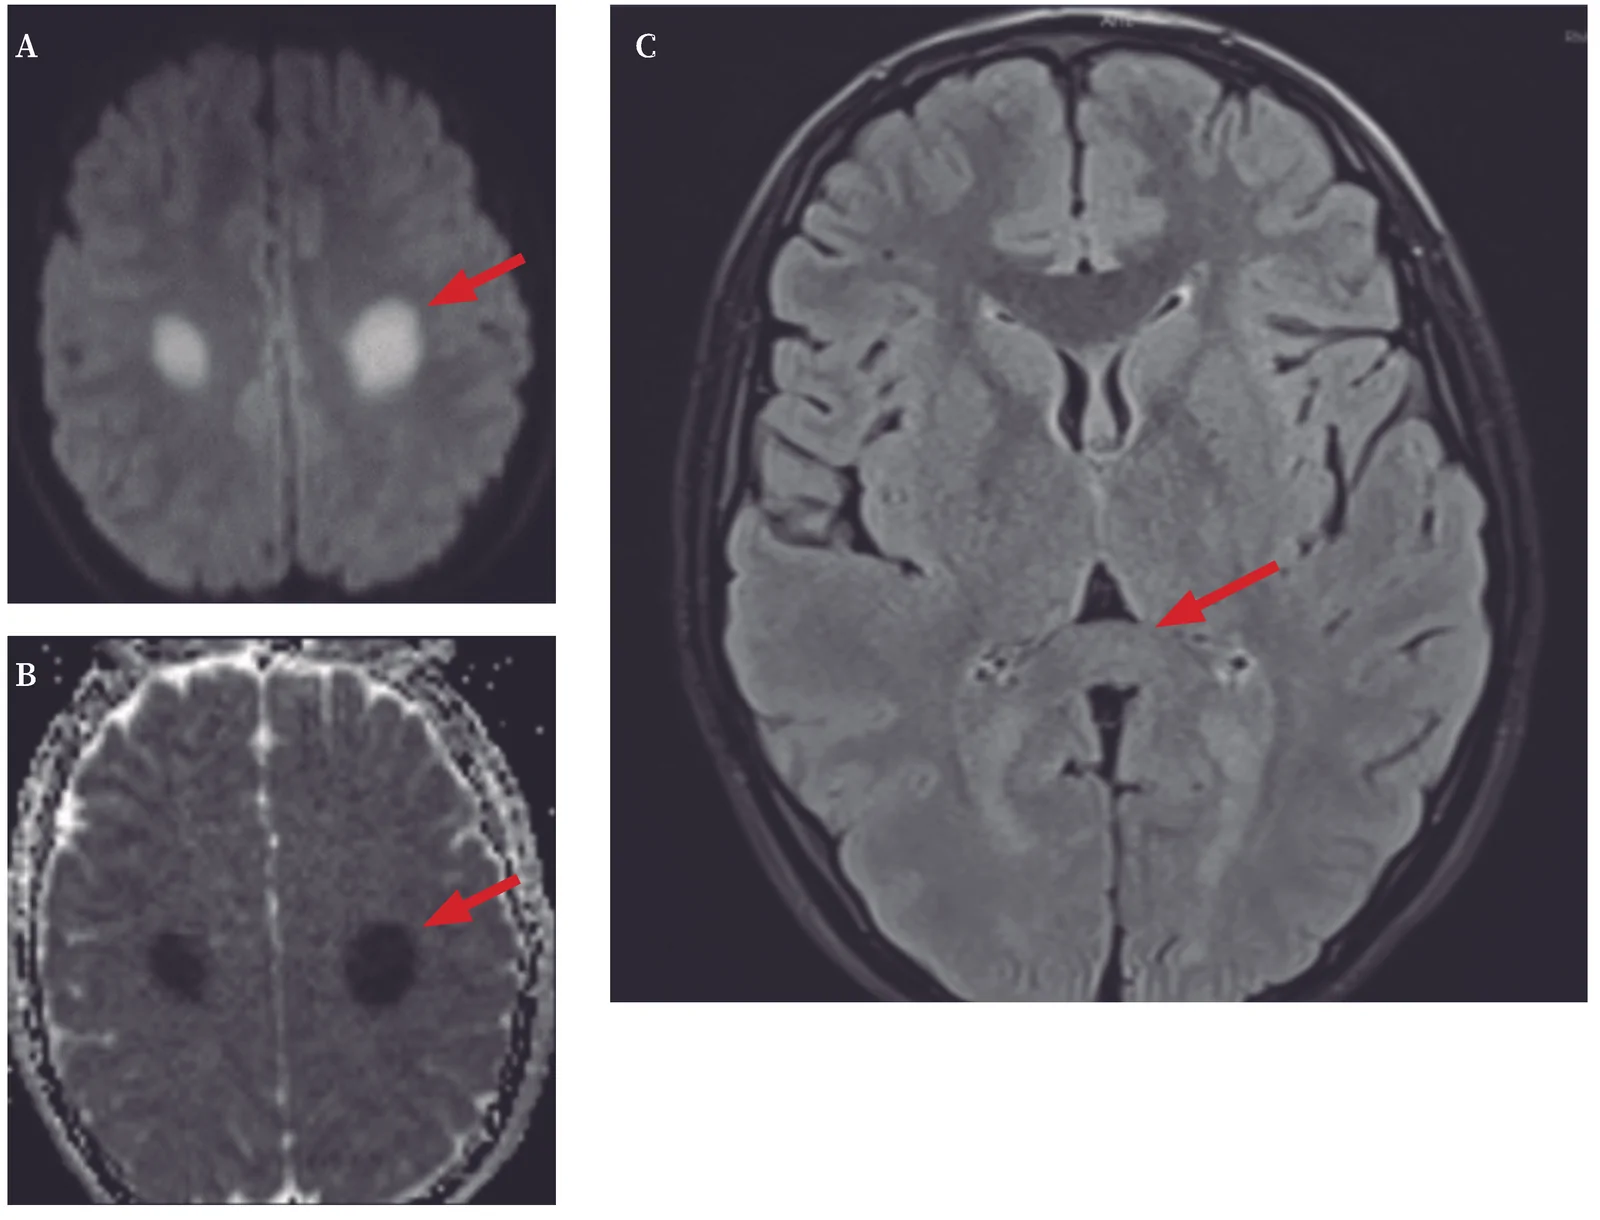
Resonancia magnética cerebral. Ambos centros semiovales (flecha) mostraron hiperintensidad en la secuencia DWI (imagen ponderada por difusión) (A), e hipointensidad en el mapa de coeficiente de difusión aparente (ADC) (B). C. La secuencia FLAIR T2 muestra alteración de señal que afecta al esplenio del cuerpo calloso (flecha).
